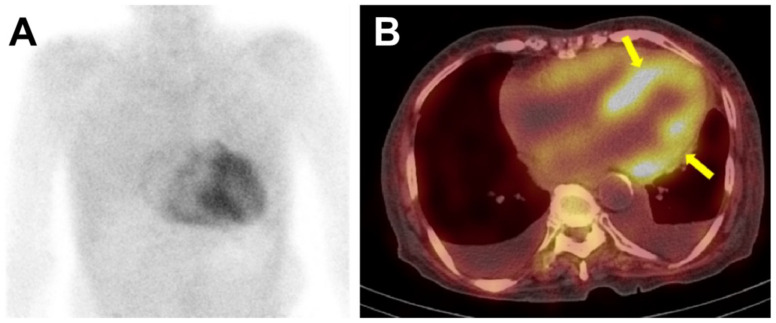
The ^99m^Tc-DPD bone scintigraphy and single-photon emission computed tomography (SPECT) of a patient with ATTR-cardiac amyloidosis. In Figure 4, the anterior planar image (panel A) showed intense, heterogeneous cardiac uptake greater than rib uptake in intensity, considered as Perugini grade 3. No abnormal radiotracer uptake was seen in other organs such as the liver or kidney. SPECT images (panel B) were acquired immediately after the planar imaging, and the radiotracer uptake could be localized to the myocardium (arrows), and not in the blood pool. Bilateral pleural effusion was also noted.
